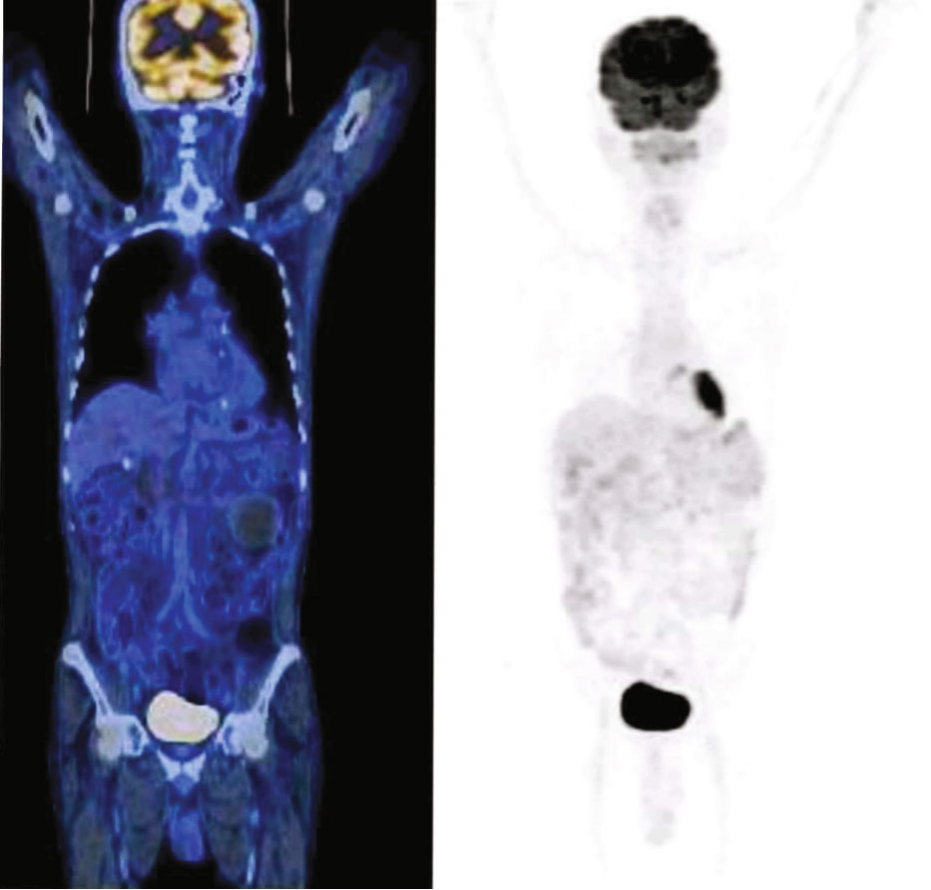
18-FDG whole body PET CT showing stable disease and metabolic activity.
